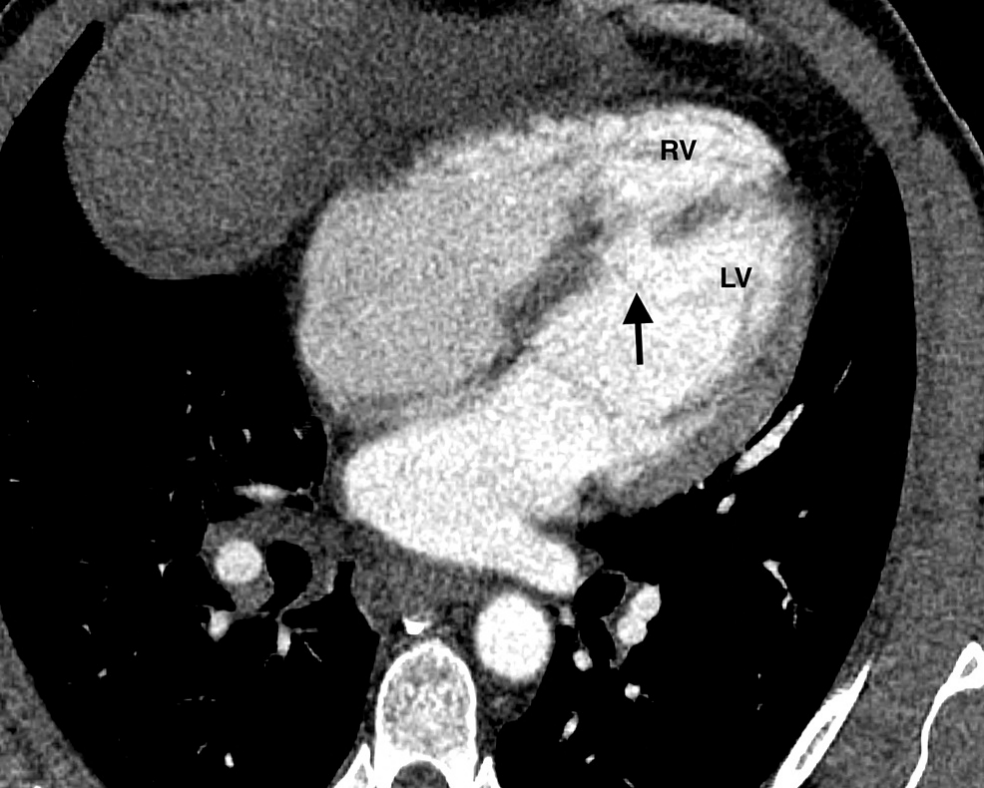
Chest computed tomography angiography demonstrating a large ventricular septal rupture in the mid inferior septal wall (arrows) with free communication between the right and left ventricles. LV, left ventricle; RV, right ventricle.
